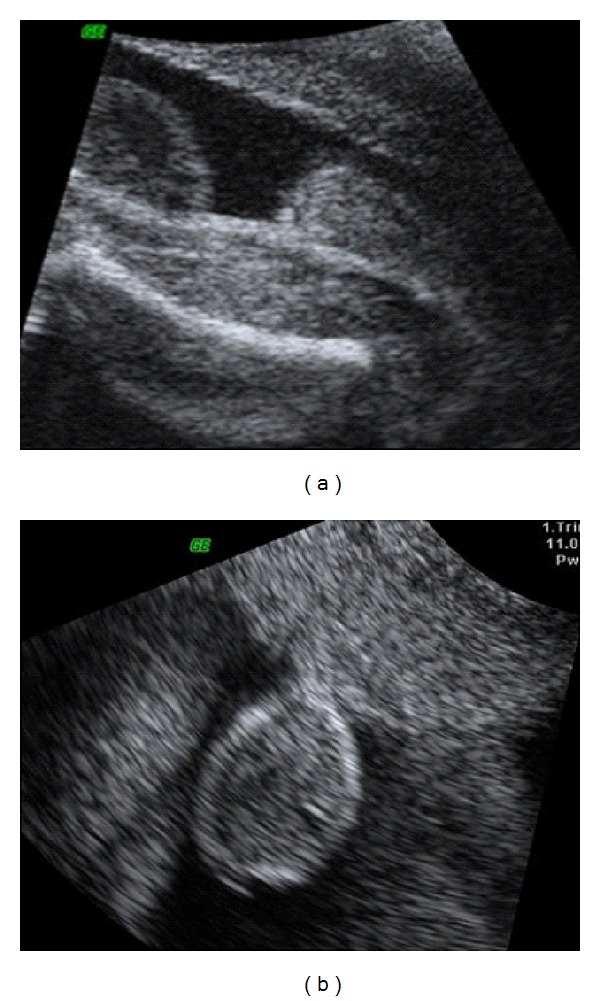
(a) and (b) Penoscrotal hypospadia assessed by two-dimensional ultrasound in the 27th week of pregnancy evaluated by abdominal approach, with clear visualization of scrotum and penis not visualized.
